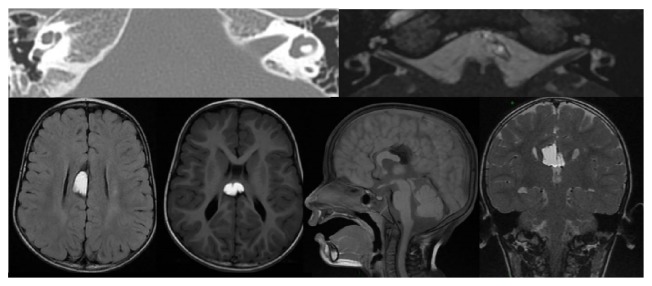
CT and MRI scans. Hypoplastic lateral semicircular canal; lipoma of the corpus callosum; agenesis of the posterior part and splenium of the corpus callosum; pellucid septum cyst; dilated cisterna magna (or cerebellomedullary cistern).
